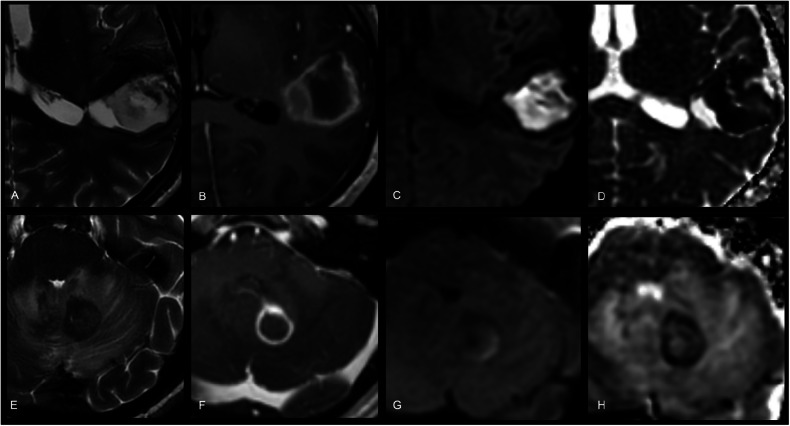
Pitfalls in the interpretation of diffusion restriction in ring-enhancing brain lesions. Top row (A-D) shows a heterogeneous T2-hyperintense lesion in the left parietal lobe (A) with the appearance of ring-enhancement on post-contrast T1-weighted imaging (B), central high signal on DWI (C), with corresponding low ADC (D) indicating restricted diffusion. While diffusion restriction suggests an abscess, the diagnosis was a lobar haemorrhage. Bottom row (E–H) shows a T2-hypointense lesion in the left cerebellum (E) with ring-enhancement on post-contrast T1-weighted imaging (F). The central portion of the lesion shows an intermediate DWI signal (G) and ADC (H). Despite a lack of diffusion restriction, the diagnosis was a toxoplasmosis abscess. Especially in abscesses from atypical organisms (e.g., toxoplasmosis and tuberculosis), diffusion is not always restricted
